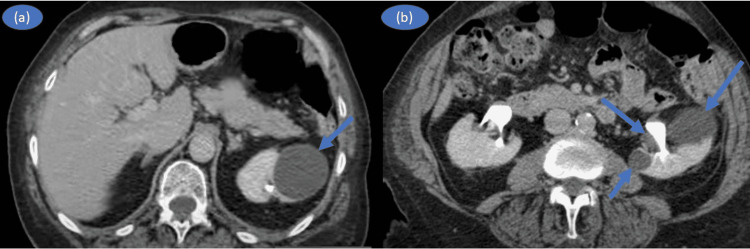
CT abdomen showing cysts at both poles of the left kidney.
